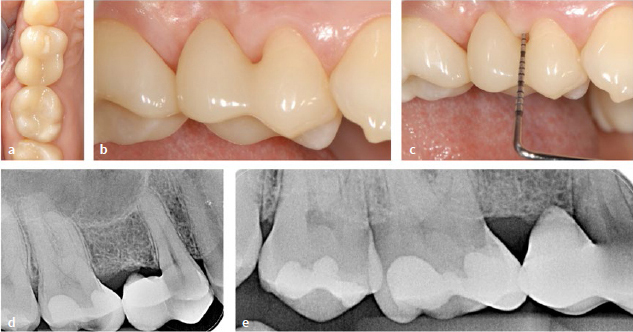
Same patient as in Fig 2, at the 1-year check-up. (a) to (c) inconspicuous clinical situation including periodontal conditions. (d) and (e) Radiographic situation.
